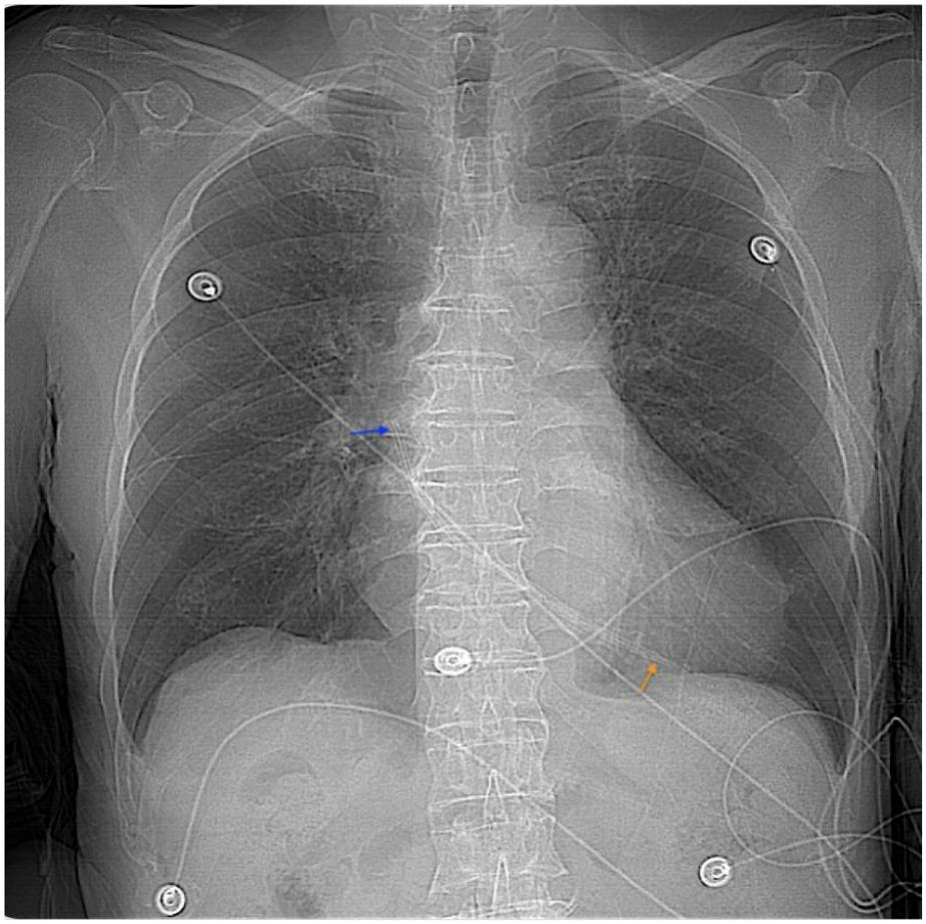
CT fluoroscopy showing the temporary dialysis catheter located in the atrium. Orange arrow point to the catheter tip, and the blue arrow point to the tail.
